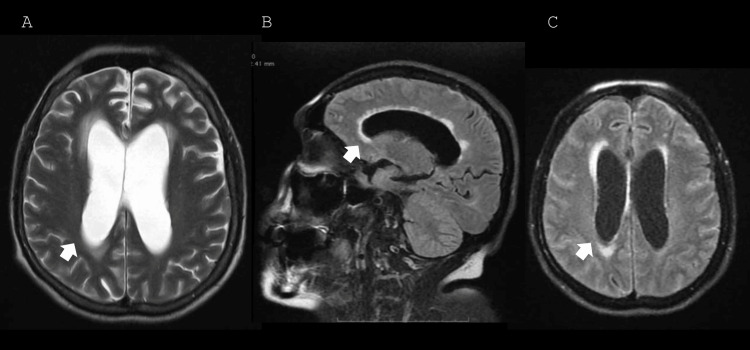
MRI of the patient in T2/FLAIR sequence showing hydrocephalus with transependymal edema. (A) Axial T2-weighted section at the level of the ventricular bodies showing ventricular dilation (arrow) and transependymal leakage, predominantly anterior and posterior. (B) Sagittal FLAIR section at the level of the ventricular bodies showing ventricular dilation and transependymal edema (arrow) extending throughout the entire ventricular body. (C) Axial FLAIR section at the level of the ventricular bodies showing hydrocephalus with transependymal edema (arrow), including extension toward the midline. FLAIR: fluid-attenuated inversion recovery
